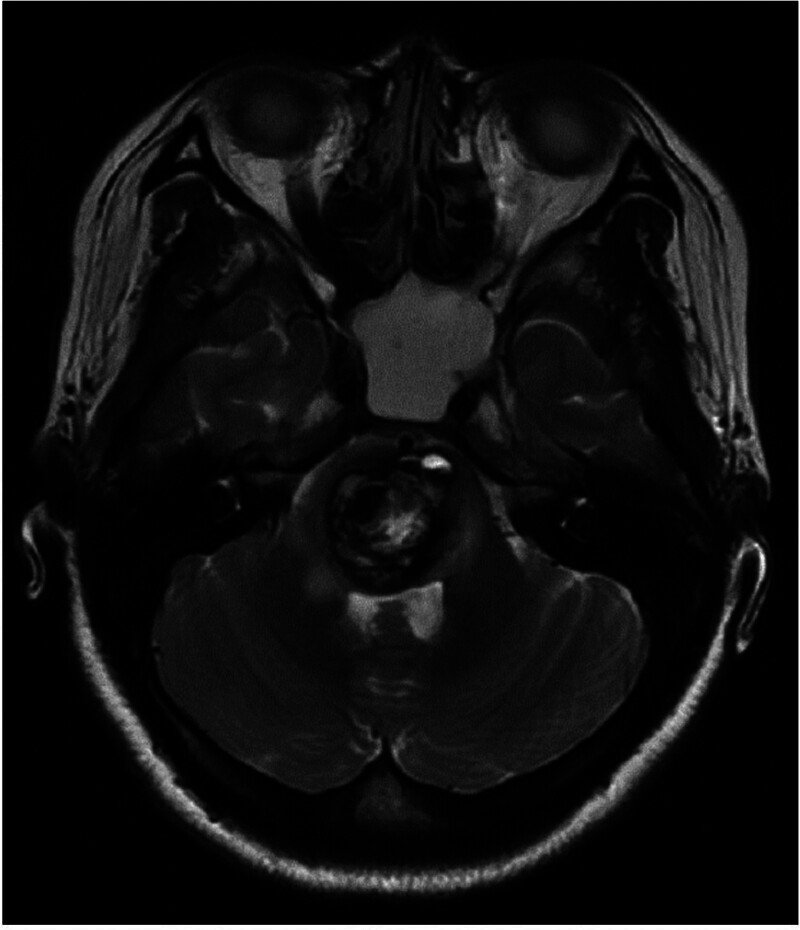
Preoperative brainstem MRI of the patient. Cerebral MRI revealed mass-like confounding of T1 and T2 signals within the pontine brain, SWAN showed hypointense signals, and central plaque hyperintense signals; little edema around lesion (SWAN: T2* weighted angiography). MRI = magnetic resonance imaging.
